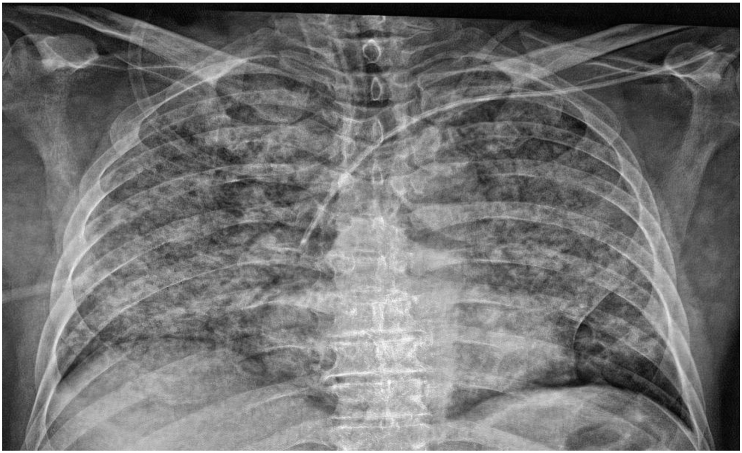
Chest X-ray showed the presence of bilateral and diffuse alveolar interstitial opacities in the two pulmonary fields.
